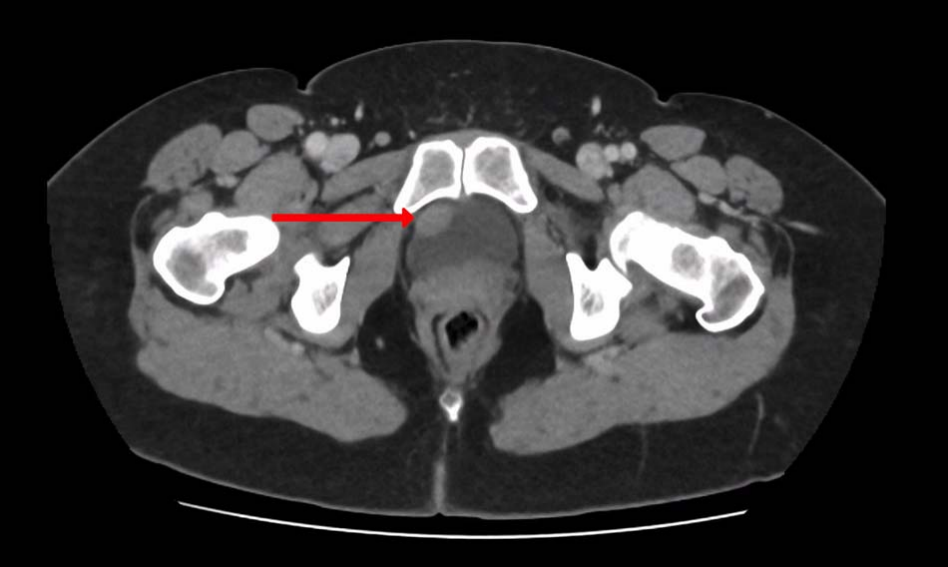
CT urogram (axial view) revealing a 1.5 cm thickening at the level of the right lateral bladder neck, and a 1-cm-soft tissue thickening at the bladder dome.
